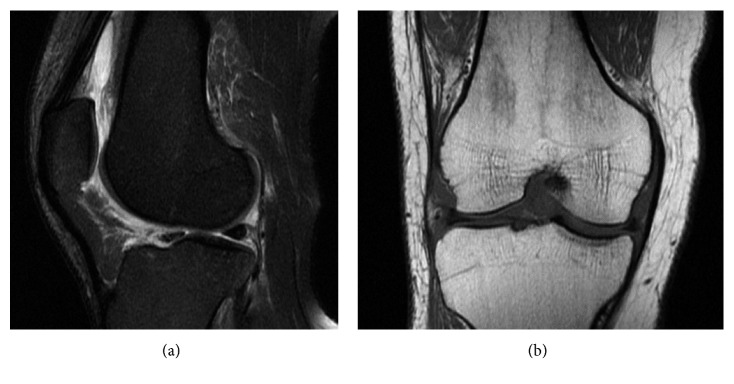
MRI of the knee in (a) T2 sagittal view and (b) T1 coronal view.
